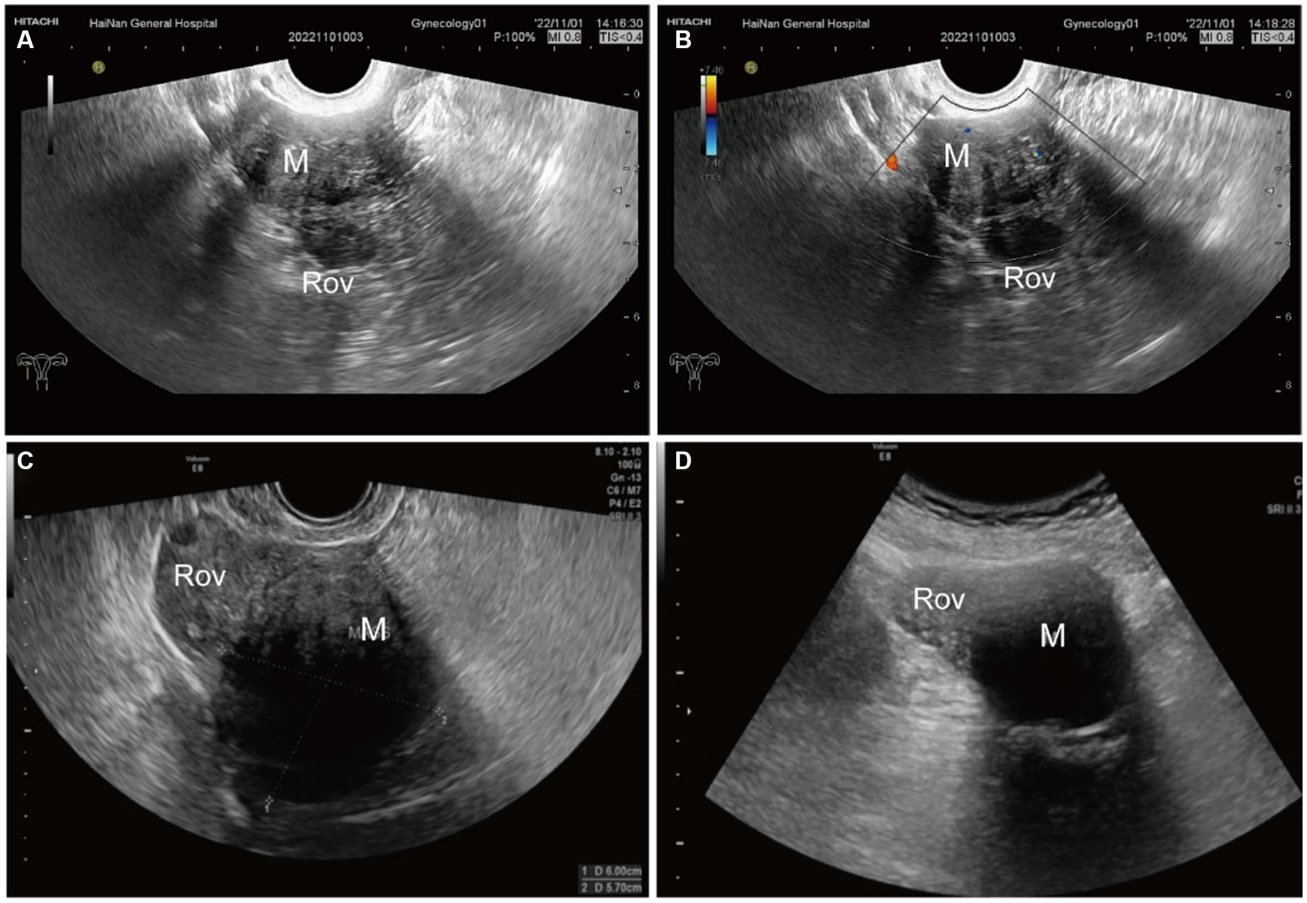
Ultrasonographic imaging of OL localized to the ovary periphery in case 6 (A,B) and case 5 (C,D). (A) A heterogeneous hypoechogenic, well-circumscribed, oval-shaped adnexal mass adjacent to the right ovary was detected by transvaginal ultrasound. The mass size was 42 mm × 29 mm. (B) Punctate blood flow signals around the mass were detected by color Doppler ultrasound. (C,D) Transvaginal ultrasound (C) and transabdominal ultrasound imaging (D) demonstrated a heterogeneous mass with more hypoechoic signal than the surrounding ovary and echo attenuation. The mass size was 69 mm × 53 mm. ROV, right ovary; M, mass.
